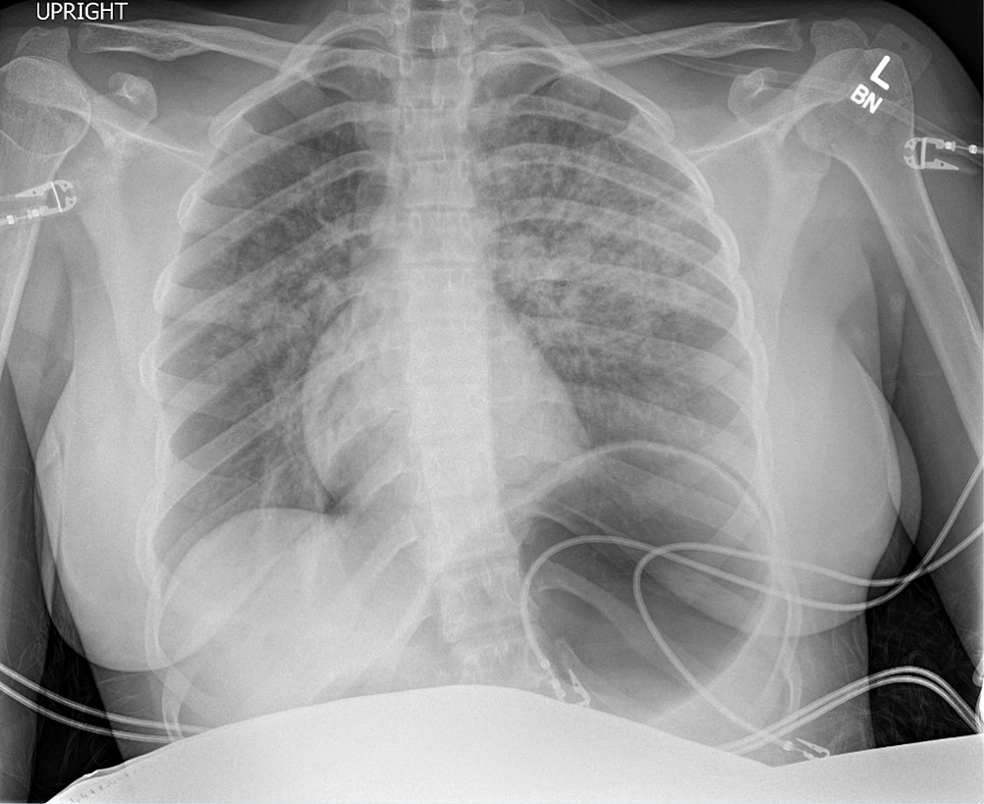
Anterior-posterior chest radiograph of the patient immediately after the surgery in the recovery unit.
